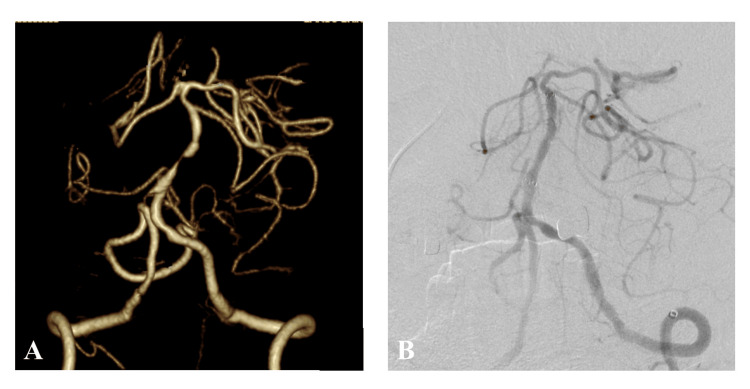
3D computed tomography angiogram (CTA) and digital subtraction angiography (DSA) of Case2 A: 3D CTA of Case 2 shows severe stenosis of the basilar artery. B: DSA obtained just after stenting of the basilar artery demonstrates a complete dilatation of the basilar artery.
